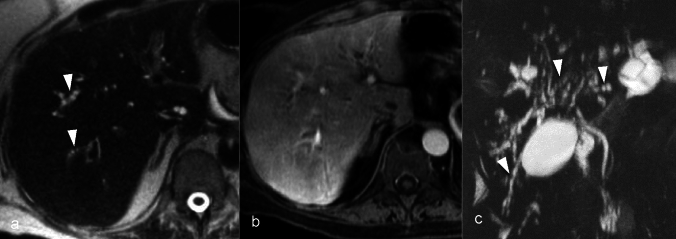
52-year-old female with metastatic clear cell RCC, treated with Ipilimumab + Nivoluman presented with acute rise in liver function test. Axial T2W MRI image demonstrates beaded appearance of the bile ducts (arrowheads, a). Post contrast venous phase T1W MRI shows heterogeneous enhancement of the liver parenchyma (image b). Magnetic resonance cholangiopancreatography maximum intensity projection shows beaded dilation of the bile ducts (arrowheads, c). Liver biopsy findings included acute cholestasis with bile duct injury and ductular reaction. The findings were consistent with a diagnosis of drug induced cholangiopathy after excluding other etiologies. The diagnosis was concordant with the clinical diagnosis of immunotherapy induced cholangitis
